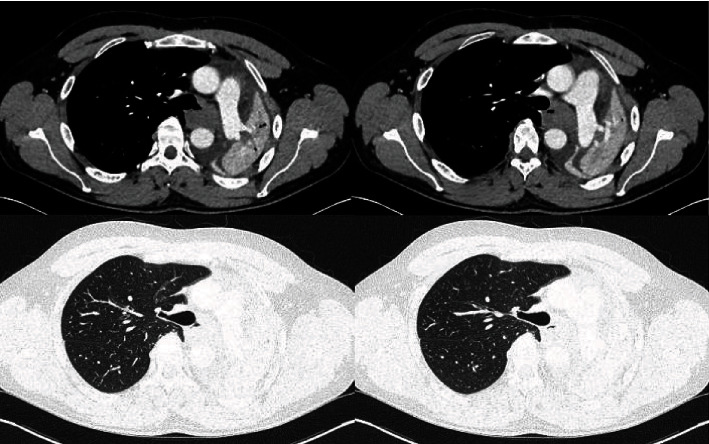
CT scan findings before the surgical procedure: a mediastinal gangliotumoral complex obstructing the left main bronchus, invasion of the carina and the lower trachea with an ipsilateral pulmonary collapse.
